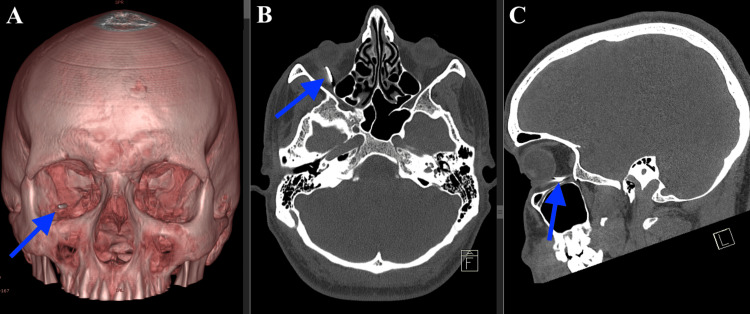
CT scan of the head CT: Computed tomography; IOFB: intraorbital foreign body; 3D: three-dimensional (A) 3D reconstruction of the CT scan showing the IOFB (blue arrow). (B) Axial plane of the CT scan showing the IOFB (blue arrow). (C) Sagittal plane of the CT scan showing the IOFB (blue arrow)
